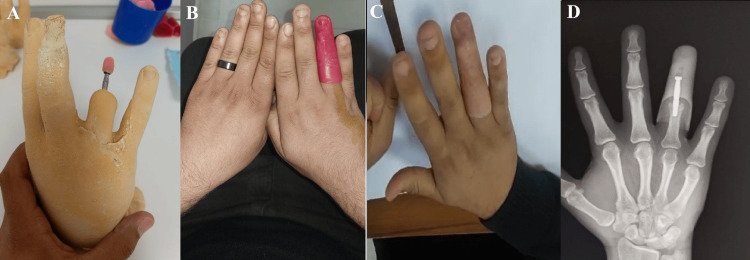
(A) Hand cast model with the abutment in position for fabrication of a retained prosthesis. (B) Initial retained prosthesis. (C) Final silicone prosthesis in situ. (D) Radiographic image demonstrating the connection between the abutment and the dental implant, with the prosthesis in place.
